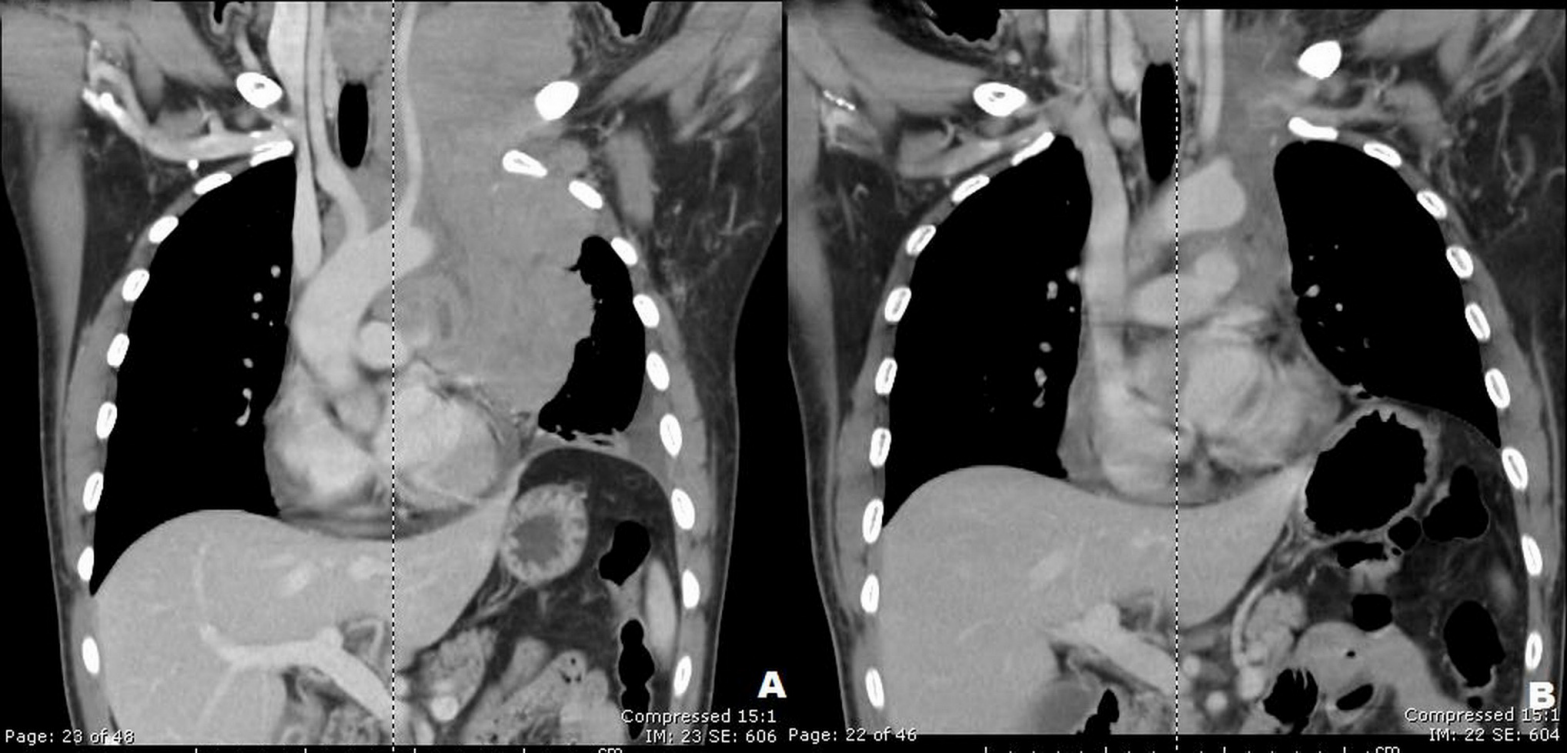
Computed tomography (CT) of the neck and chest showed a large lobulated heterogeneous-enhancing mass with central necrosis at the anterior and the middle mediastinum with some parts extending into the left anterior neck (A). Significant improvement of lymphomatous involvement at the prevascular region was seen after eight cycles of CHOP chemotherapy (B). CHOP, Cyclophosphamide (Cytoxan), Hydroxyrubicin (Adriamycin), Oncovin (Vincristine), Prednisone.
